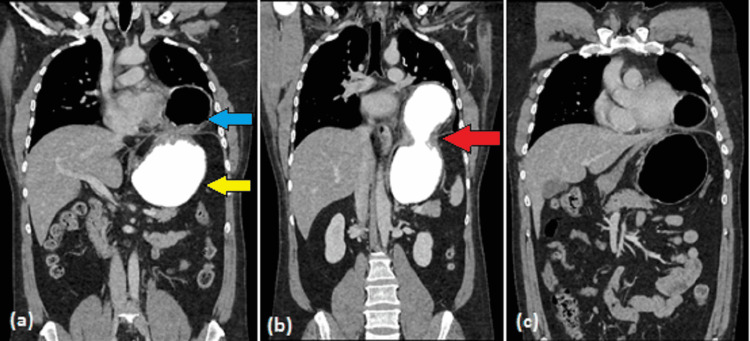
CT scan coronal images with intravenous and oral contrast The images show a left diaphragmatic defect (red arrow) with herniation of the proximal stomach (blue arrow) into the left hemithorax. The distal part of the stomach is seen in its anatomical position in the left upper abdomen (yellow arrow) CT: computed tomography
